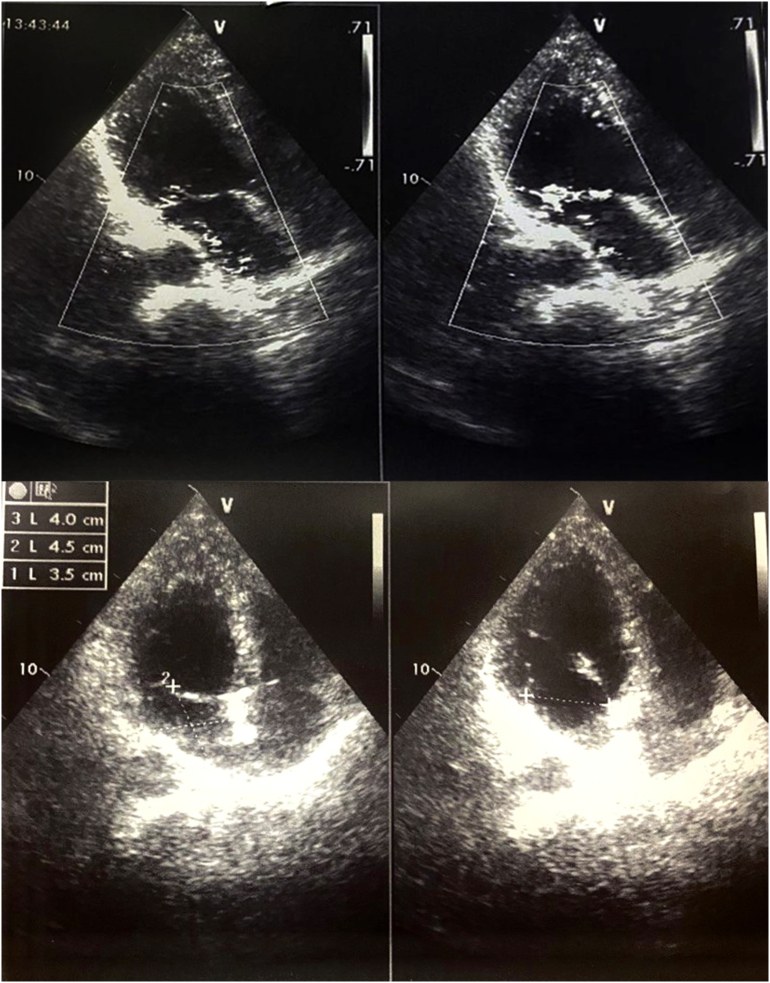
Showing transthoracic echocardiogram which demonstrated severe al regurgitation and anterior leaflet A2 prolapse from ischemia and degenerative changes. The annulus measuring 35 mm (normal from 28 to 32).
